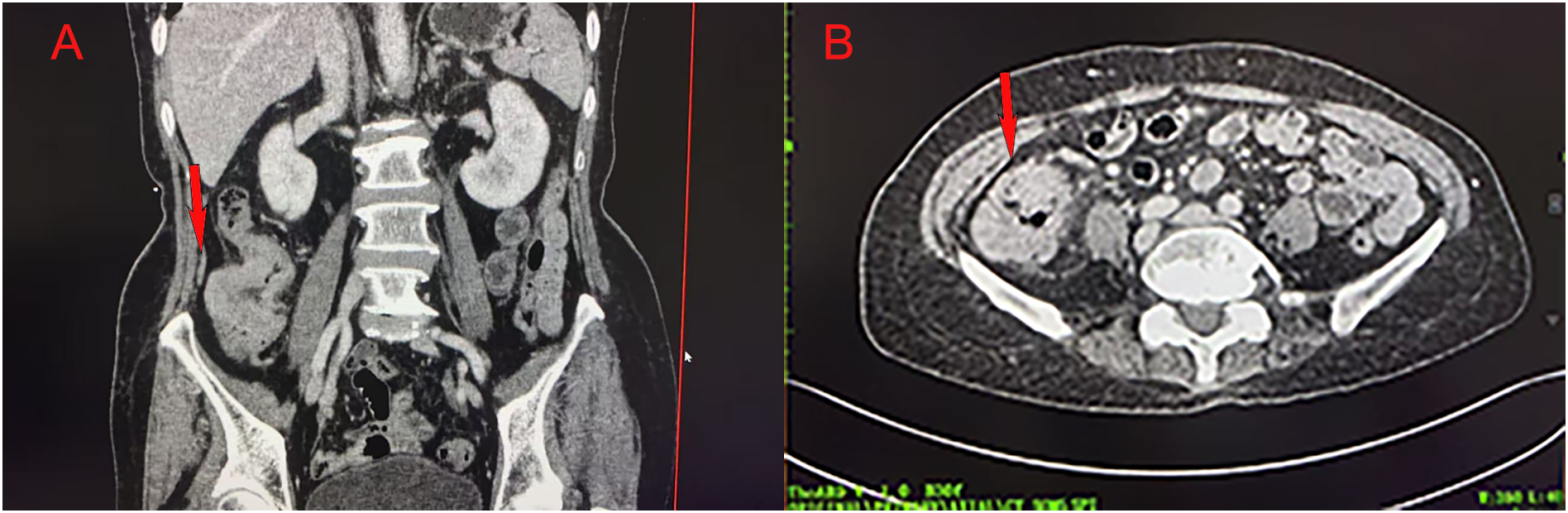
Abdominal CT showing an ascending colon mass in coronal (A) and axial (B) views.
